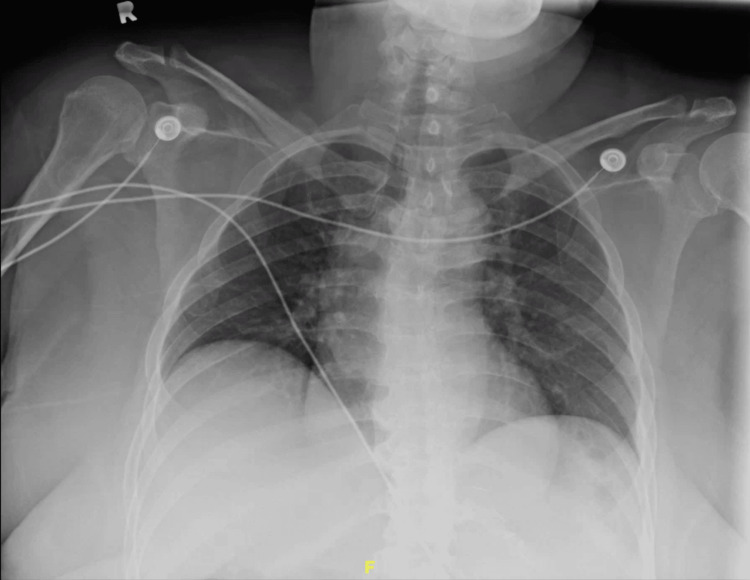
Xray of the chest showing normal anatomy
